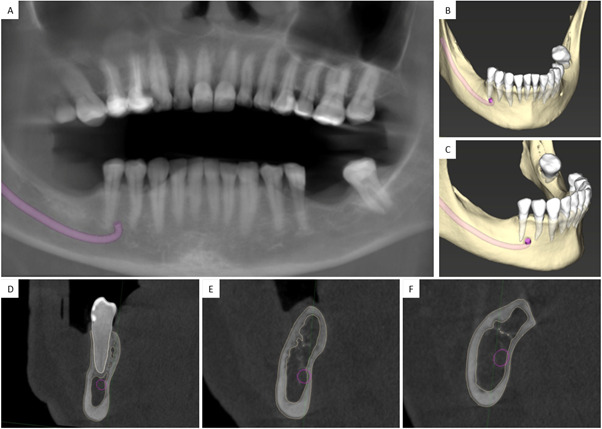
A) Reconstrucción panorámica de CBCT de control. B) Vista frontal de la reconstrucción 3D de CBCT con un trazado del NDI. C) Vista lateral de la reconstrucción 3D de CBCT con un trazado del NDI. D) Corte transversal de la pieza 15 en relación con el nervio dentario inferior. E) Corte transversal. F) Corte coronal de tomografía de control de la zona quirúrgica con adecuada neoformación ósea, sin compromiso del NDI.
